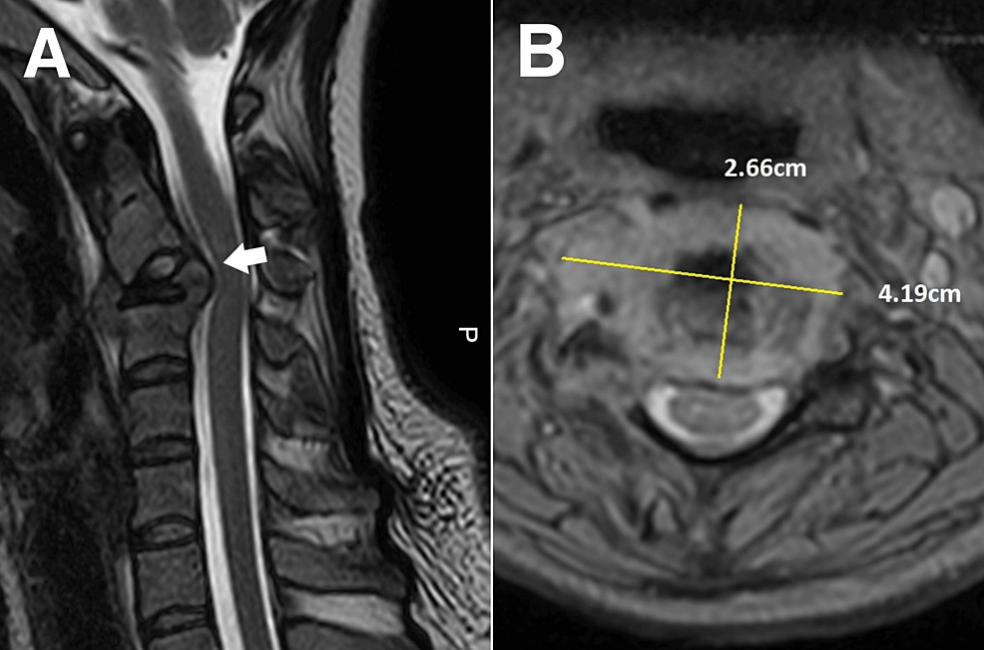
Cervical magnetic resonance image A) Vertebral plana in the C3 body with a T2 hyperintense lesion in C3. Mild cord compression at the C3 level. B) An overall size of 2.66 × 4.19 cm
